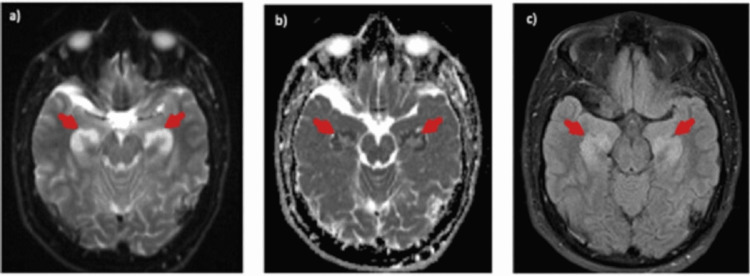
Brain MRI in Case 2, axial views. a) Diffusion-weighted imaging b) apparent diffusion coefficient sequences showing diffusion restriction with corresponding c) T2 fluid-attenuated inversion recovery hyperintensities in bilateral hippocampi (arrows).
